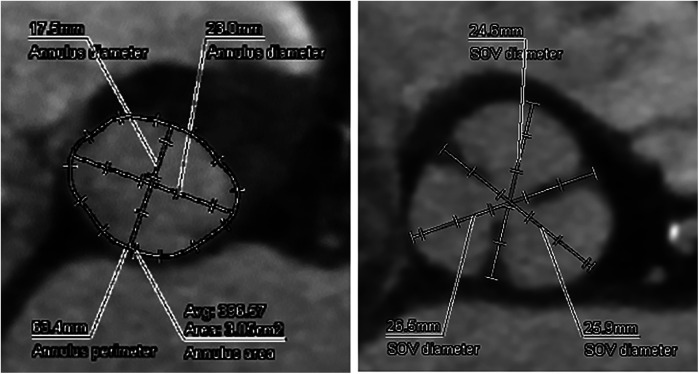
Preoperative CT analysis of the A-valve complex. Annulus perimeter was 63.4 mm, annulus area was 305 mm^2^, suggesting a narrow annulus.
